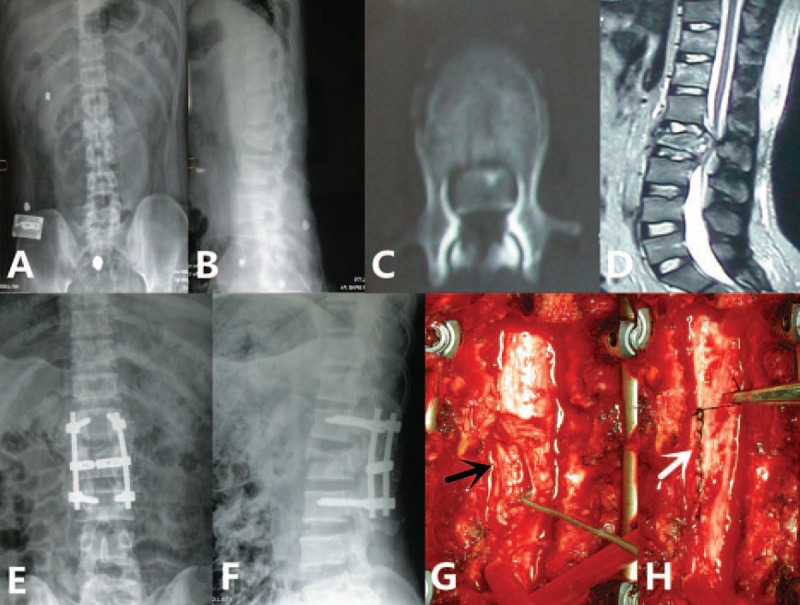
A 32-year-old male patient with L2 burst fracture, with ASIA C neurological impairment. (A and B) preoperative anteroposterior and lateral radiographs showed a L2 burst fracture; (C and D) computed tomography scan and magnetic resonance imaging showed bone fragment intrusion and severe spinal cord compression; (E and F) 3d postoperative anteroposterior and lateral radiographs showed vertebral height was restored, spinal cord was decompressed, and angular deformity was corrected by using posterior pedicle screw fixation; (G and H) intraoperative photograph showed dural laceration, herniated cauda equina rootlets (the black arrow) and the sutured dural sac (the white arrow).
